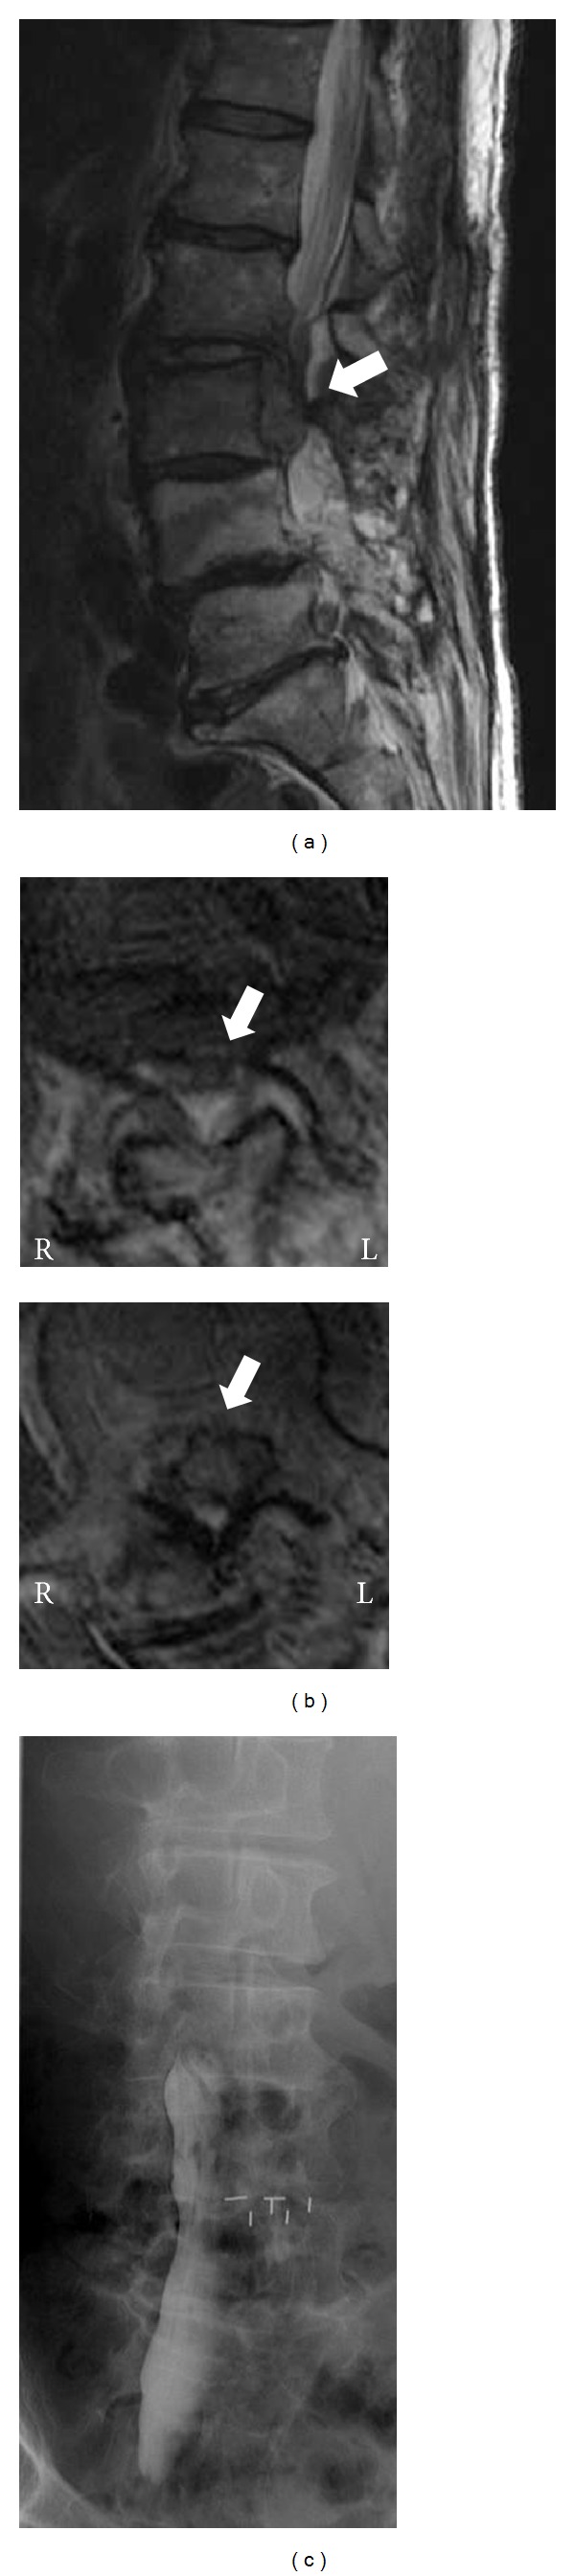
(a) Sagittal T2-weighted magnetic resonance image at the time of admission demonstrating a large descending disc hernia from the L2/3 to L3 levels. (b) Axial T2-weighted image of the L2/3 level (upper) and L3 vertebra level (lower) showing a hernia (arrow) compressing the spinal cord posterolaterally. (c) Myelogram obtained in a head-lowering prone position showing obstruction at the L3 vertebra level.
